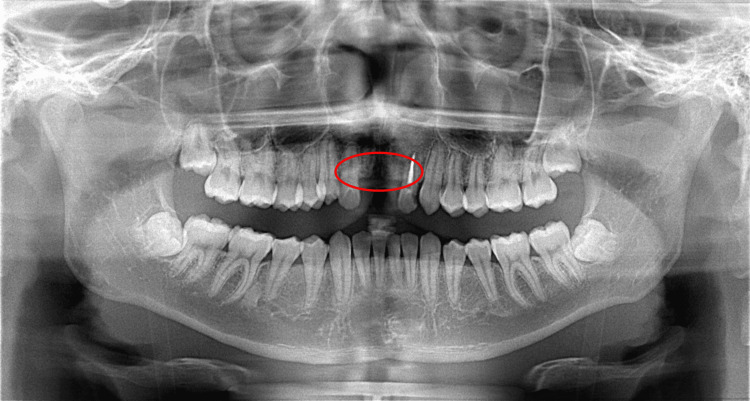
Pre-treatment panoramic radiograph The bone volume of the missing incisor area was reduced (indicated by the encircled region).
